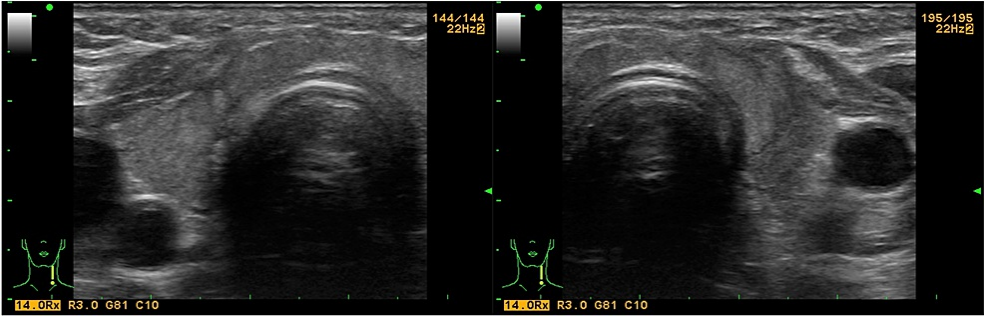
Ultrasound of the thyroid after six courses of chemotherapy The thyroid parenchyma has inhomogeneous structure. The formation seen at baseline is not visible.
